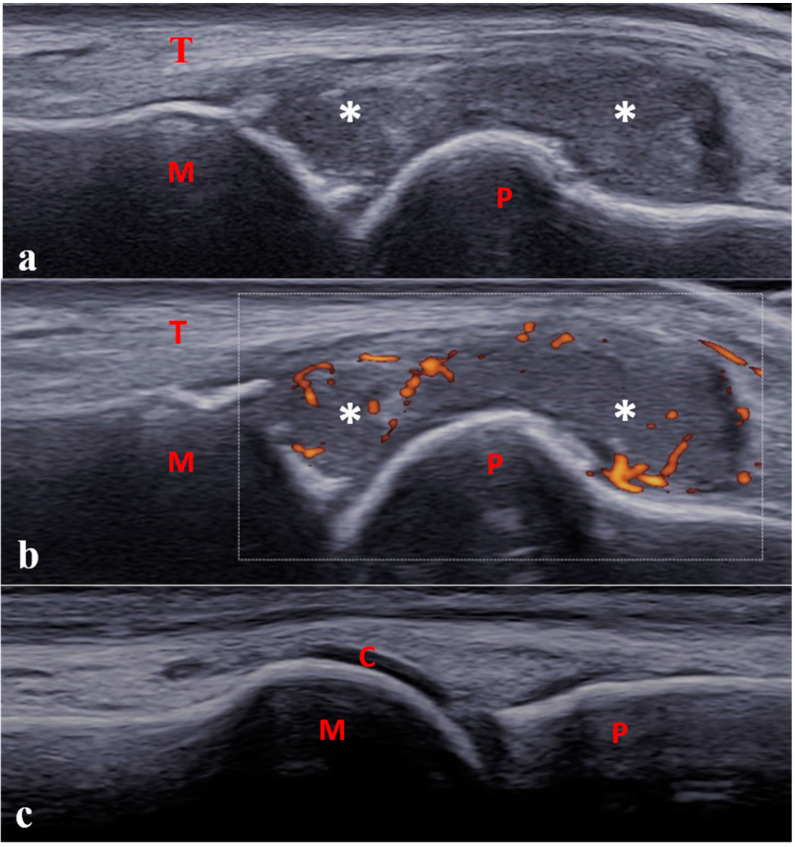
Longitudinal dorsal scan of one metatarsophalangeal joint in a 13-year-old boy with enthesitis-related arthritis. (a,b) Severe synovitis (B-mode grade 3) with moderate power Doppler activity (<30% of the synovial hypertrophy surface, grade 2). (c) Physiological aspect of the contra-lateral joint. C: cartilage; M: metatarsal; P: proximal phalanx; T: tendon; * Synovitis.
